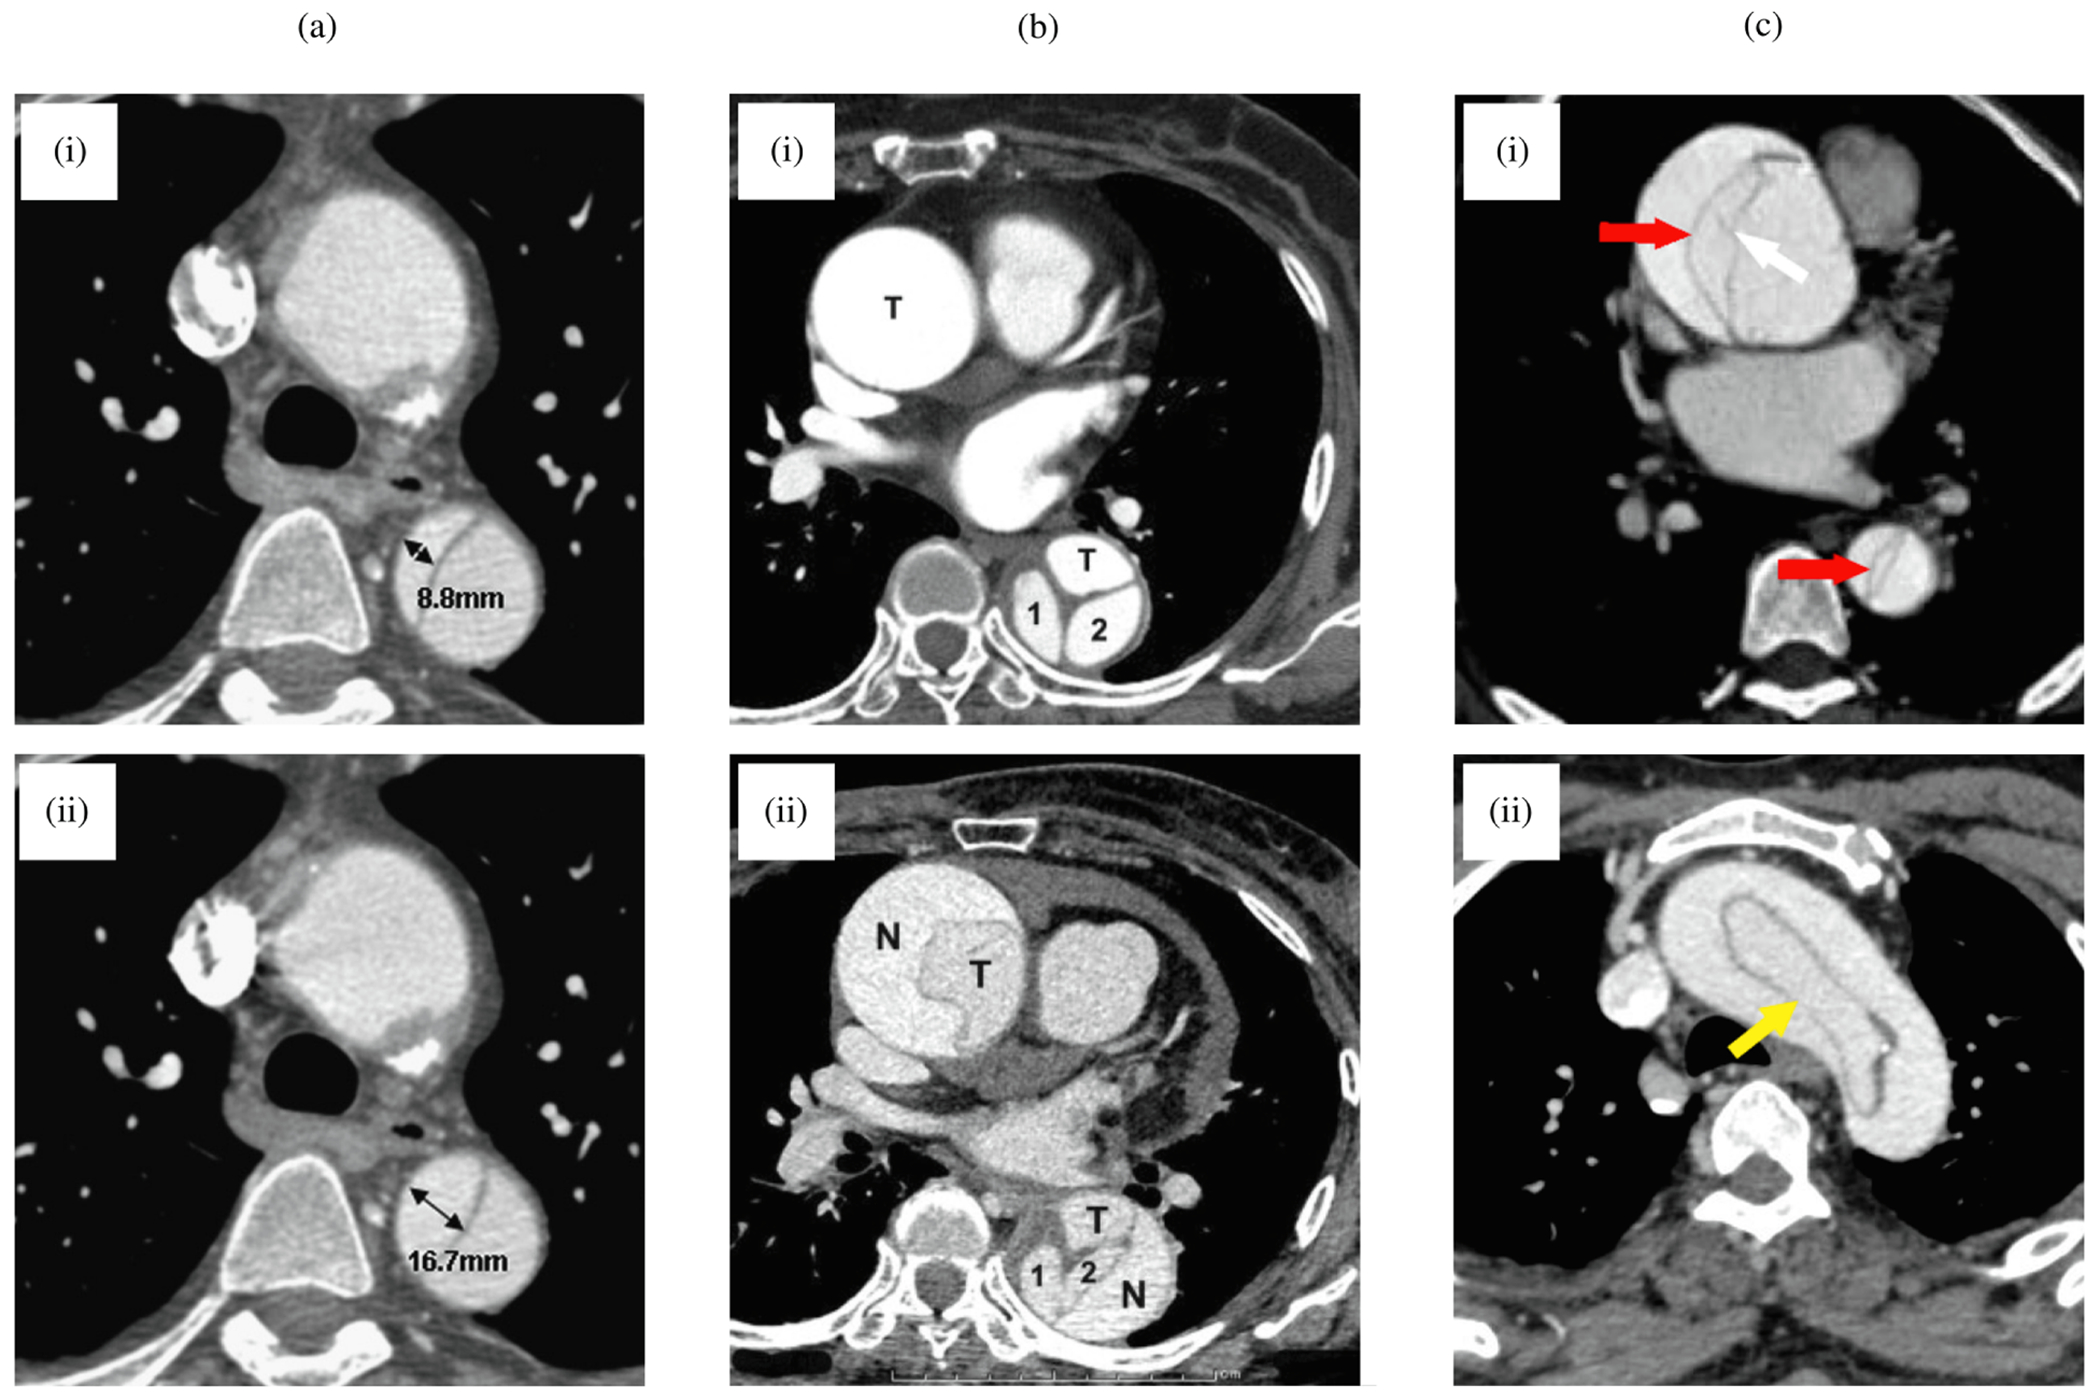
(a) A representative CT image shows a patient with a dissection flap oscillation amplitude of 7.9mm, which is due to different luminal pressures, with (i) a minimal short axis diameter of 8.8mm (ischemic configuration) and (ii) a maximal short axis diameter of 16.7mm (benign configuration) measured at the end of the aortic arch. (b) Another CT image shows (i) a ‘triple-barreled’ dissection in the descending aorta (‘T’: true lumen; ‘1’ and ‘2’: false lumina), which later developed into (ii) a ‘quadruple-barreled’ dissection (‘N’: new false lumen). (c) In addition, a CT image shows (i) the dissection flap (red arrow) in the ascending and descending aorta with linear hypodense structures within the false lumen (white arrow) resembling the dissection flap, known as ‘cobweb sign’, and (ii) a type A dissection with intimointimal intussusception, in which the circumferential dissection flaps with oval morphology (‘windsock sign’) involve the aortic arch (yellow arrow). Figure (a) is adapted from [278] with permission from Elsevier, while (b) and (c) are from Awal et al. [279] (licensed under CC-BY-NC 4.0), and (d) is from Sueyoshi et al. [280] with permission with Elsevier.
